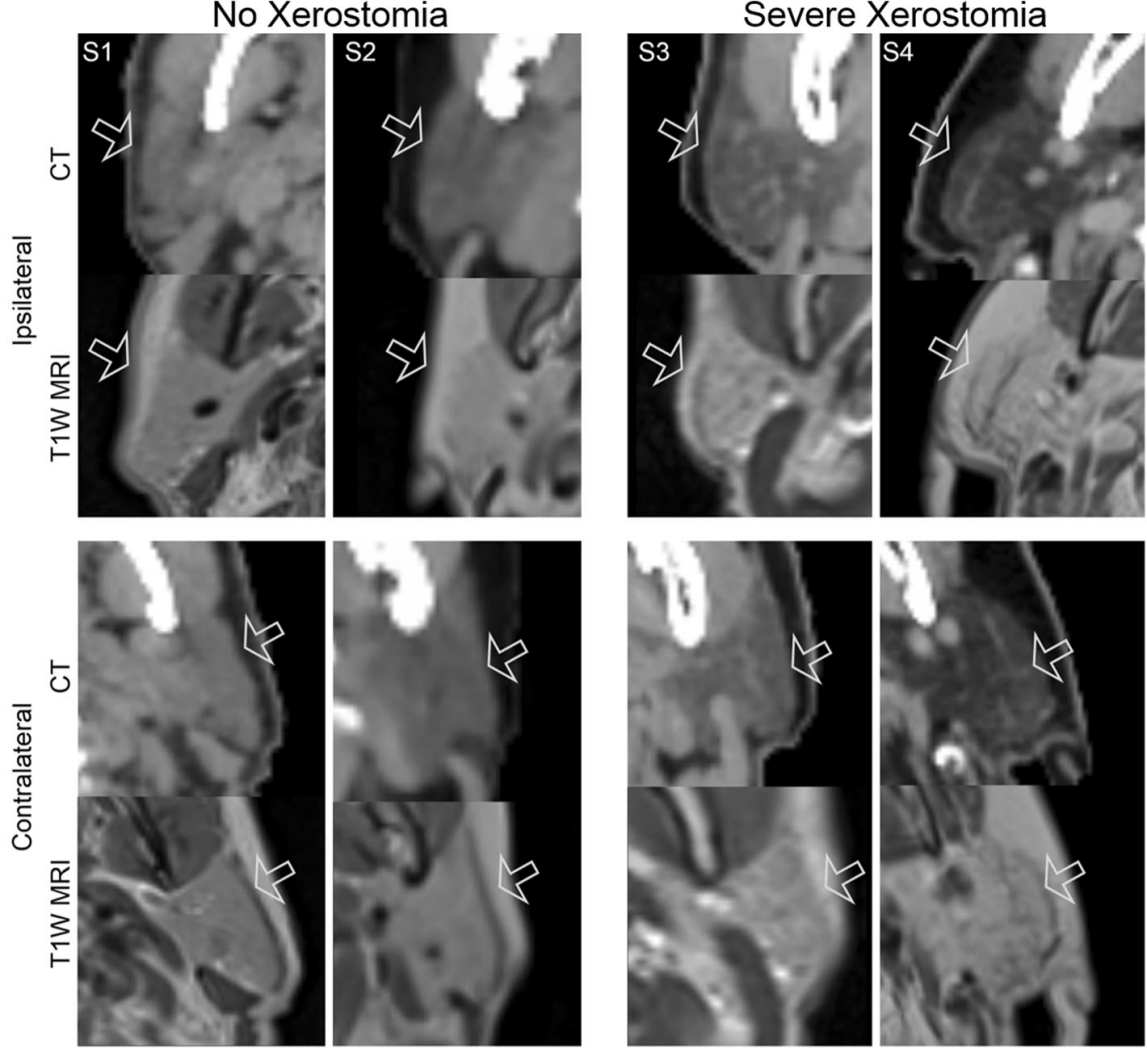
CT and MR images of representative patients’ parotid glands. From left to right: S1: 57-yr old male with squamous cell carcinoma of the nasopharynx, S2: 82-yr old female with melanoma of nasal cavity, S3: 61-yr old male with squamous cell carcinoma of the nasopharynx, and S4: 69-yr old male with carcinoma ex pleomorphic adenoma of eye. Images are displayed using the same window and level
